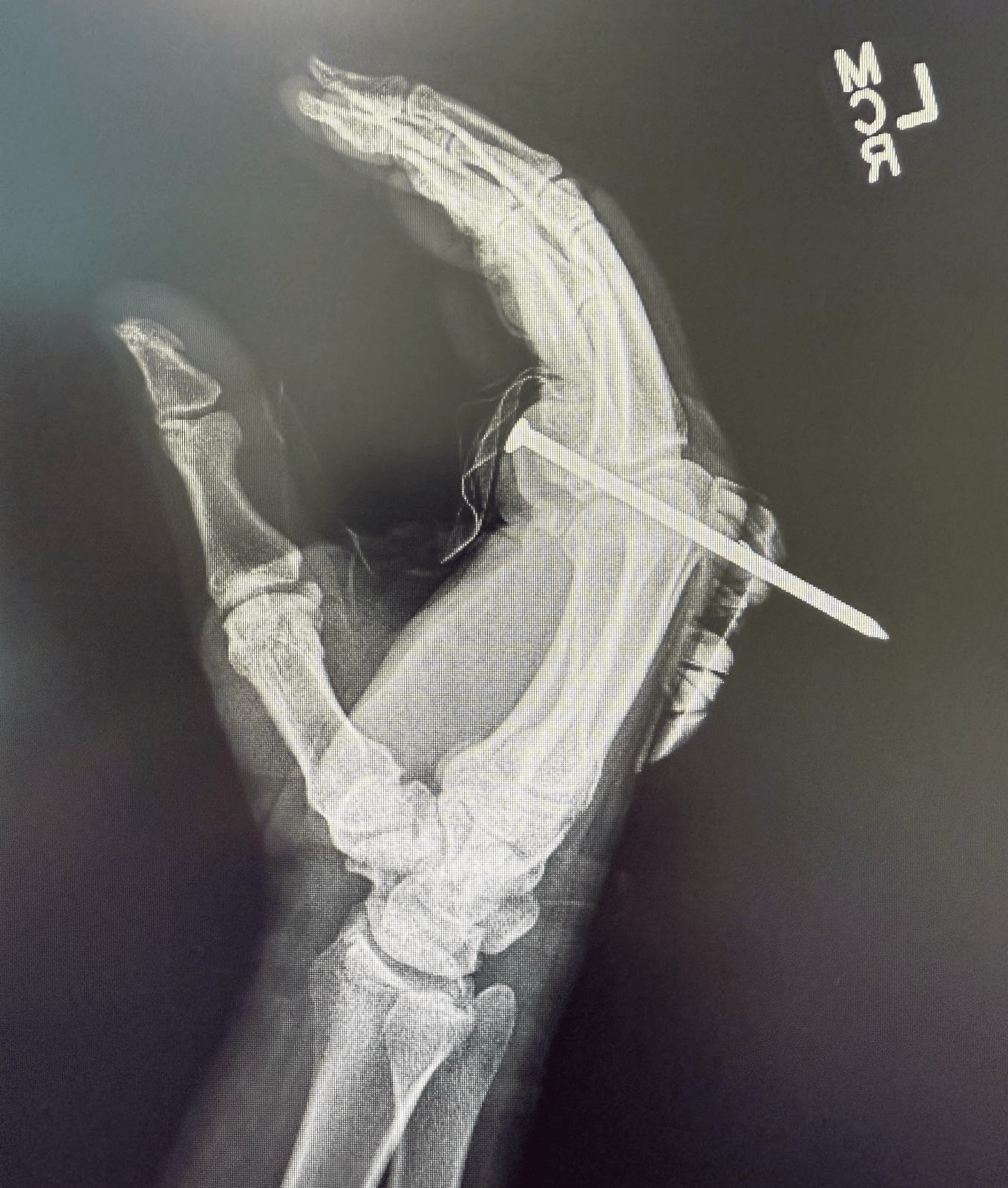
Lateral X-ray image X-ray image highlighting the orientation of the nail.
